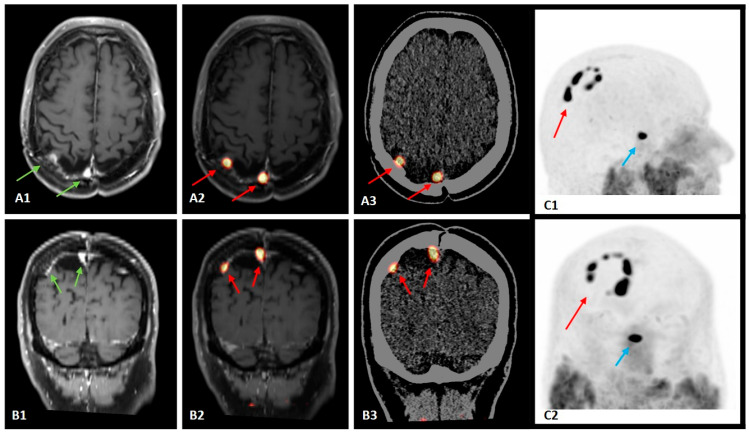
A 60-year-old male with recurrent atypical meningioma, previously treated with surgery (6 years before) and radiation therapy for a local recurrence (5 years before), underwent segmental brain somatostatin receptor positron emission tomography/computed tomography (SR-PET/CT) for restaging after magnetic resonance imaging (MRI) findings suggested a possible recurrence. SR-PET/CT was performed 60 min after the injection of 200 MBq of Gallium-68 DOTATATE (a radiolabeled somatostatin analogue), and PET images were also fused with the recent MRI images. SR-PET image analysis was performed by using qualitative criteria: areas of increased radiopharmaceutical uptake compared to the background, excluding the sites of physiological radiotracer uptake, were considered abnormal. Furthermore, semi-quantitative PET image analysis was performed by using the maximal standardized uptake value (SUV_max_). Axial and coronal T1-weighted MRI images (A1,B1), fused PET/MRI images (A2,B2), fused PET/CT images (A3,B3) and maximum intensity projection PET images (lateral view (C1), anterior view (C2)) revealed a novel “rosary sign” with multiple adjacent areas of focal abnormal radiopharmaceutical uptake (red arrows) along the resection margins of the previous surgical site in the right parietal region, corresponding to hyperintense lesions at T1-weighted MRI (green arrows), while the pituitary showed physiological tracer uptake (blue arrows). The “rosary sign” was particularly relevant because it highlighted multiple foci of abnormal radiopharmaceutical uptake linked together, suggesting disease recurrence and confirming the suspicion raised by MRI, as well as identifying a higher number of lesions. The highest SUV_max_ of the meningiomatous lesions was 16.9. Due to the high SR expression demonstrated by SR-PET/CT with this novel imaging pattern and considering progressing/recurrent disease after multiple treatments, this patient was addressed with peptide receptor radionuclide therapy (PRRT) [1,2,3]. SR-PET/CT is indicated for the differential diagnosis of brain lesions suspicious for meningiomas, delineation of meningioma extent, detection multifocal disease/extracranial metastases, and monitoring of disease progression or diagnosis of recurrence, playing a crucial role in these clinical assessments [1,2,4,5,6,7,8,9]. PRRT is a well-tolerated treatment option for both meningiomas and neuroendocrine tumors with high somatostatin receptor expression. Patients with progressive/recurrent/treatment refractory meningiomas and increased SR expression are ideal candidates for PRRT if a previous SR-PET/CT demonstrates high SR expression [1,2,3].
